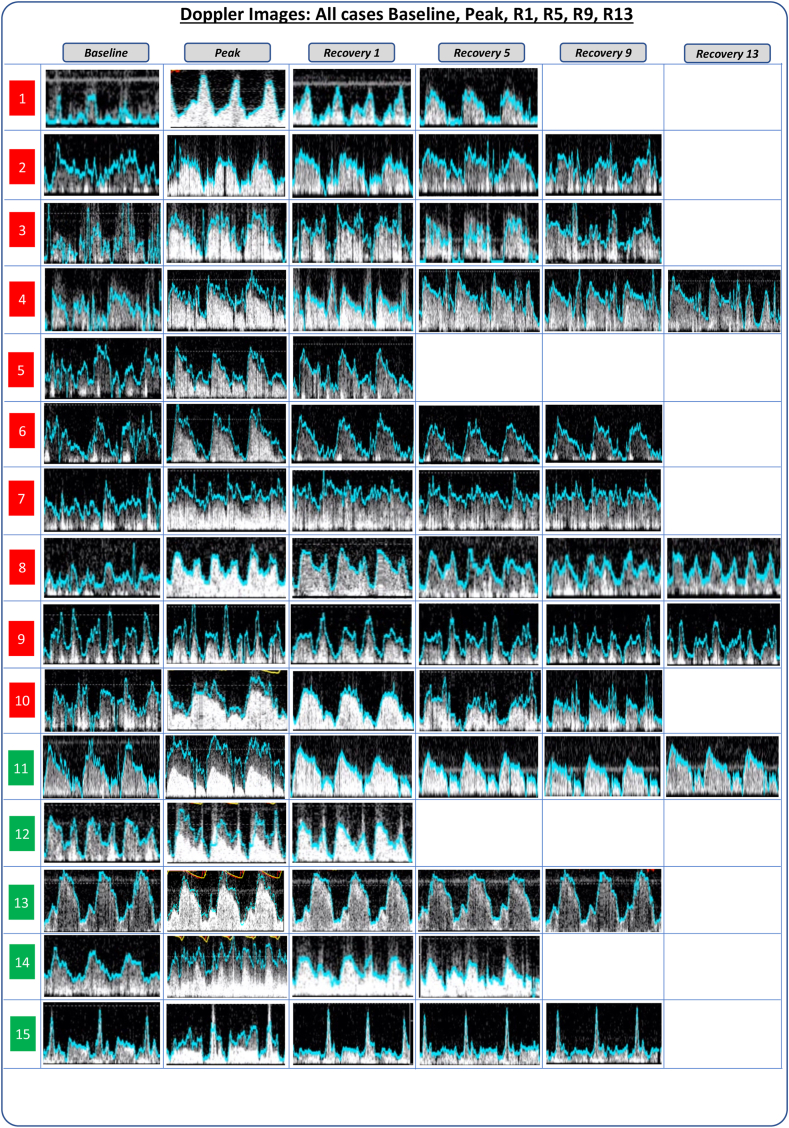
Doppler images of all included vessels demonstrating the effect of microbubble ultrasound contrast. The first 10 rows (red numbers) show those judged to have poor quality signals, whereas the next 5 rows (green numbers) show those judged to have good quality signals. After the administration of microbubble contrast, one can appreciate an improvement in the quality of the Doppler signal, with a marked improvement in signal density. In addition, particularly in the group with poor quality signals, there is a marked reduction in the variability of the tracked velocities (light blue line).
